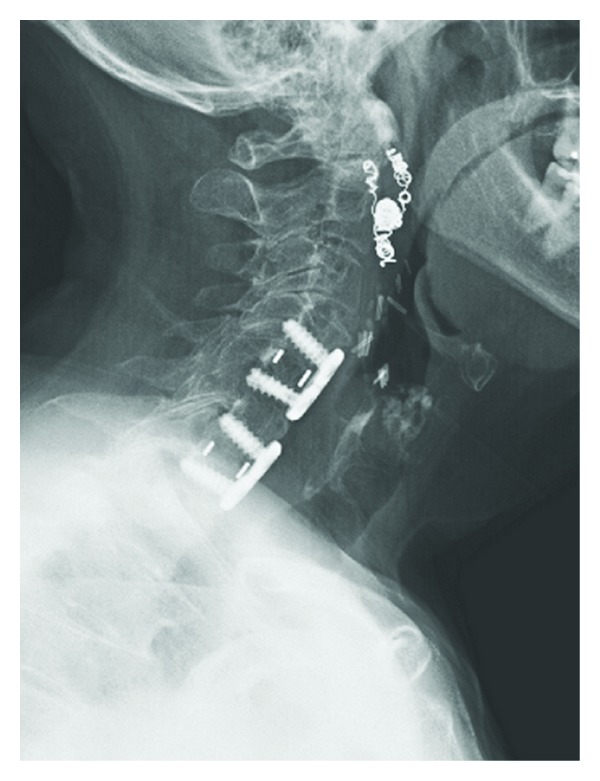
Postoperative lateral radiograph showing fixation at 9 months. (Please note: clips and coils postembolization of the occipital and external carotid arteries apparent in the anterolateral left neck.)
